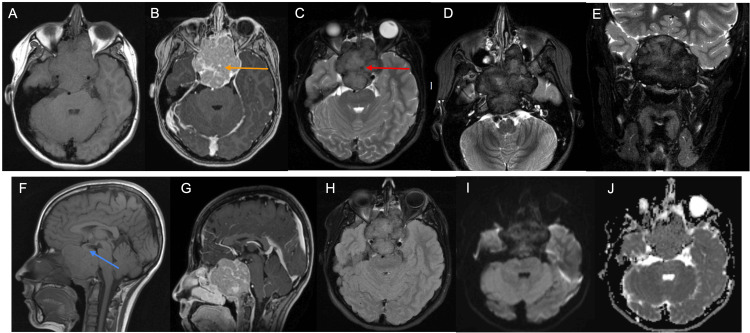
T1-weighted images in axial plane (A) before and (B) after gadolinium demonstrate a T1 isointense mass centered at the clivus with heterogeneous enhancement. (C) T2-weighted images in the axial plane at the same level demonstrate a T2 hypointense focus (red arrow) likely representing a flow void corresponding with the focus of enhancement on the postcontrast images (orange arrow). The T2-weighted images in the (D) axial plane at a more inferior slice and in the (E) coronal plane demonstrate diffuse hypointense signal within the mass and a marked T2 hypointense rim. T1-weighted images in the sagittal plane (F) before and (G) after gadolinium demonstrate superior displacement of the pituitary (blue arrow) without invasion. (H) FLAIR images in the axial plane demonstrate isointense to hypointense signal. (I) Diffusion-weighted and corresponding (J) ADC images demonstrate no diffusion restriction. FLAIR: Fluid-attenuated inversion recovery, ADC: Apparent diffusion coefficient.
